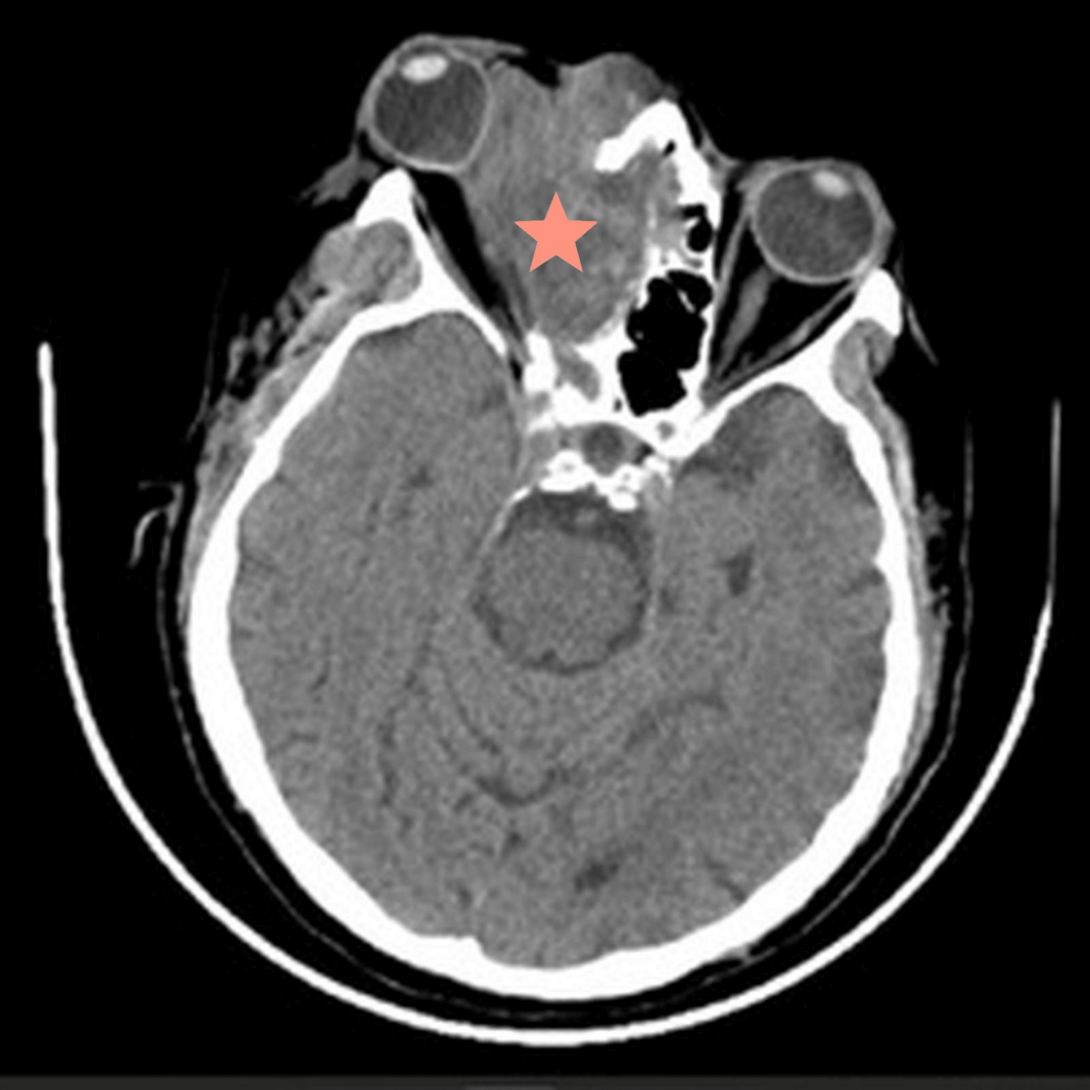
Axial brain CT showing extension of the mass into the right orbital cavity (star).
